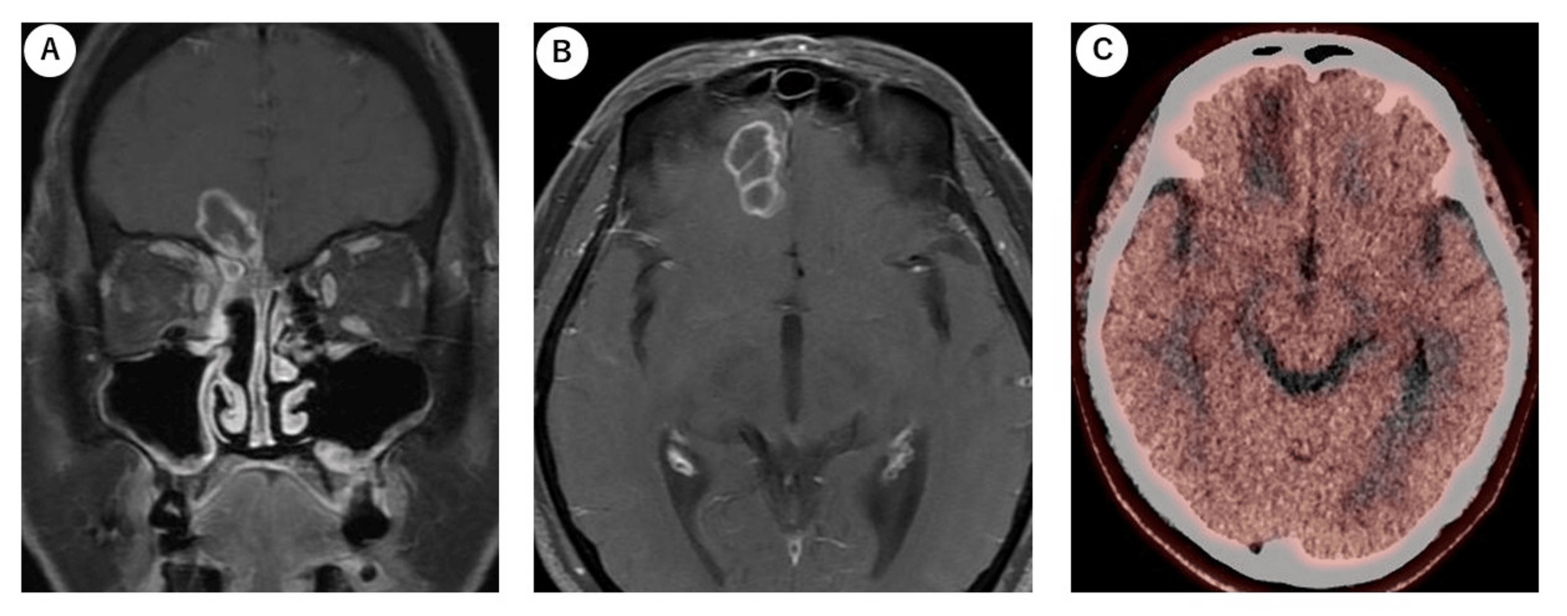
MRI and MET PET imaging after six months A, B: coronal and axial MRI images C: MET PET imaging. Very slight accumulation was observed with a mean standardized uptake (SUVmean) of 1.51 in the tumor and 1.4 in normal brain tissue. MET: 11C-methionine; PET: positron emission tomography
